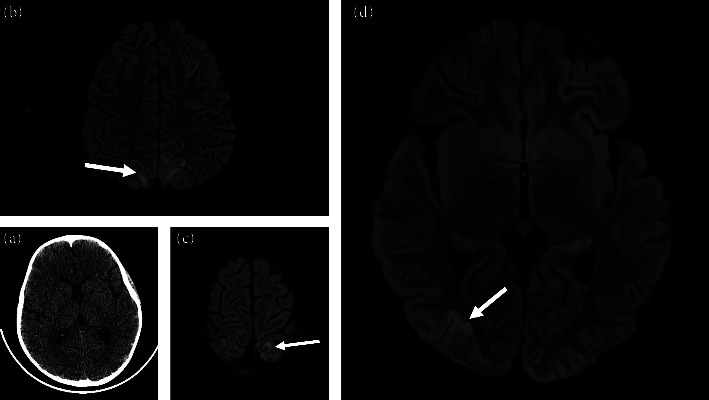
CT and MRI radiographs in a patient presenting with PRES. Following seizure-like activity, an initial CT without contrast (a) showed no acute pathology. A follow-up MRI under sedation (b–d) revealed abnormal, symmetrical signal hyperintensities in the cortex and subcortical white matter of the parietal and occipital lobes on T2-weighted-fluid-attenuated inversion recovery consistent with PRES.
